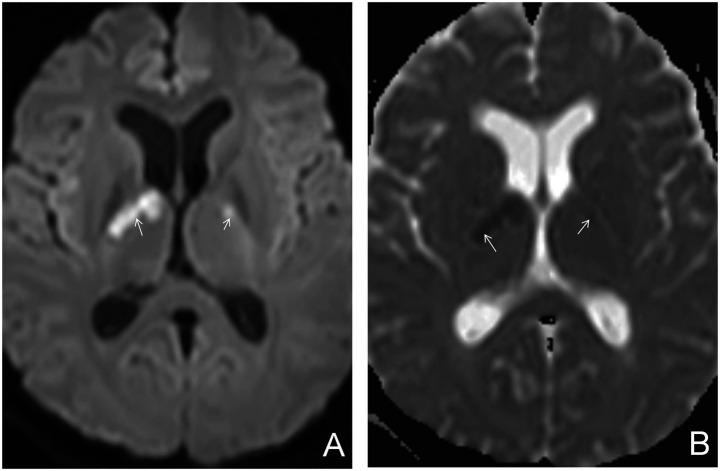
Parenchymal tuberculous granulomas with concurrent cerebral infarction. (A) DWI (*B* = 1,000) showing multiple hyperintense lesions in the bilateral basal ganglia (white arrows), indicating acute cerebral infarction. (B) ADC map showing hypointensity in the bilateral basal ganglia (white arrows).
